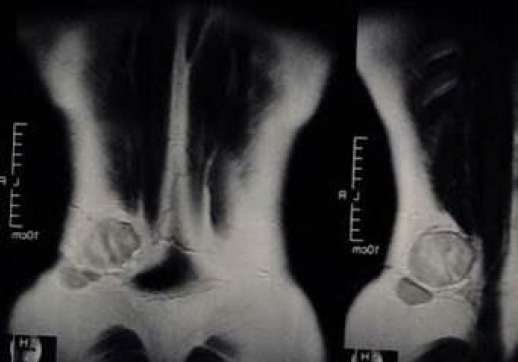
Pre-operative MRI picture.
